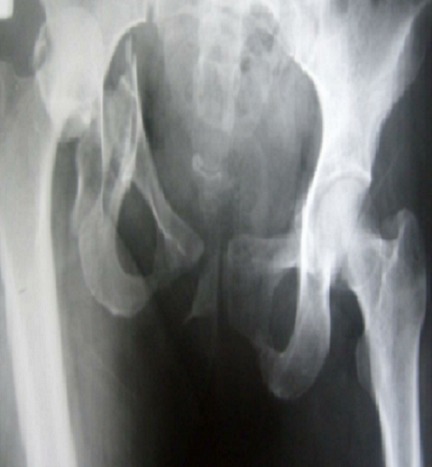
Radiographie du bassin de face montrant une fracture transversale du cotyle droit
